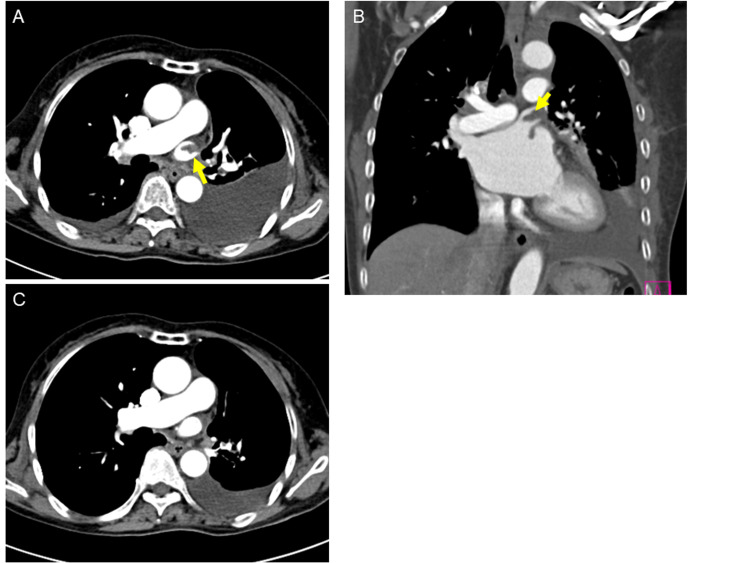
Contrast-enhanced computed tomography (CECT). (A, B) Arrows indicate thrombus formation in the left superior pulmonary vein stump on CECT seven days after onset. (C) The thrombus dissipated two months after the cerebral infarction.
